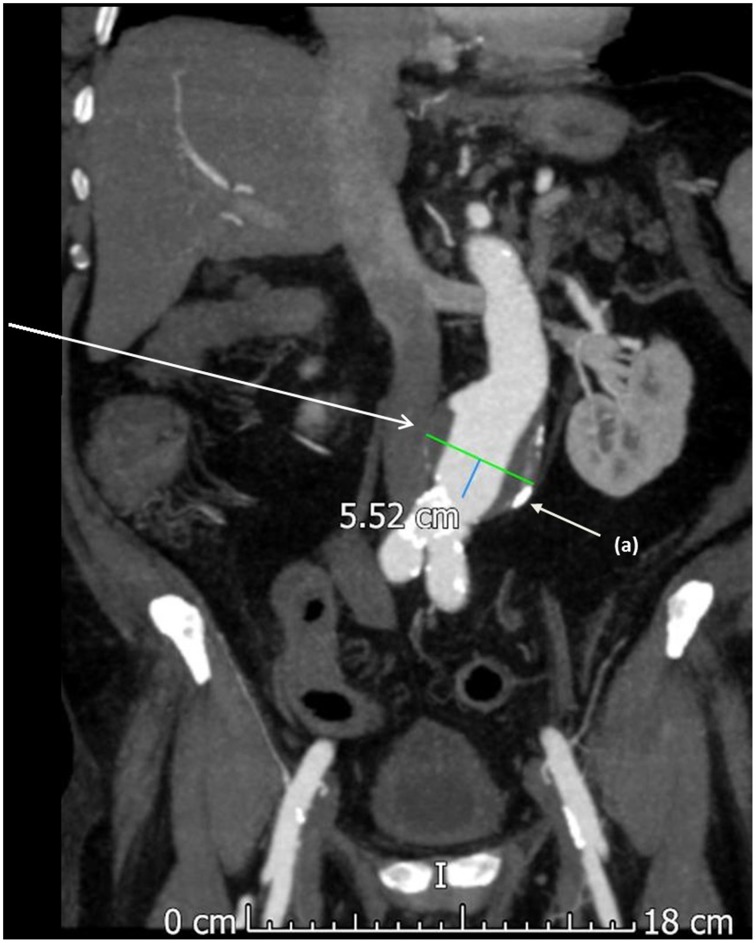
A computed tomography (CT) scan with contrast showing an abdominal aortic aneurysm. This aneurysm was asymptomatic and found during routine surveillance scanning of a 67 year old male. Calcified atherosclerotic plaque is noted on the aneurysm wall and in the distal aorta (a).
